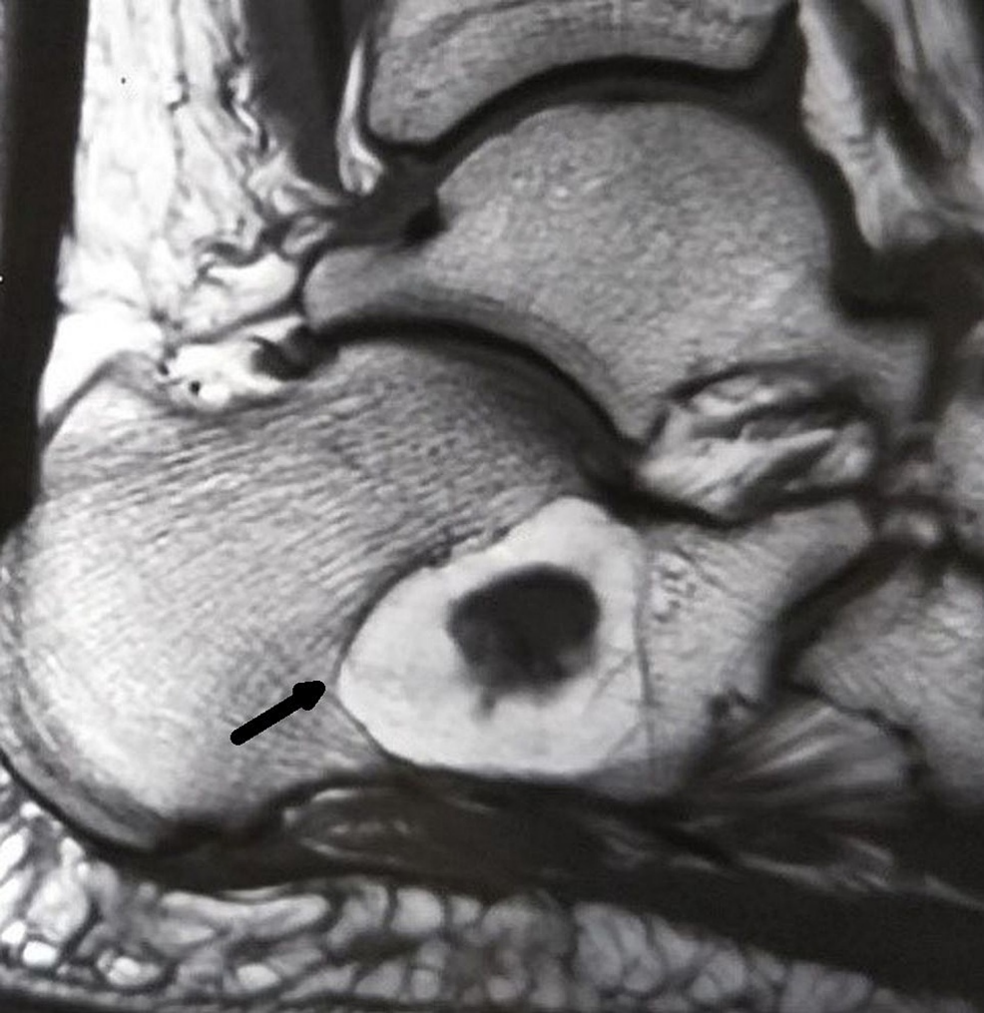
Magnetic resonance imaging of the right ankle at presentation The T1-weighted sagittal image demonstrated a 3 x 2.5 cm lytic lesion in the body of calcaneum (black arrow), with hyperintense signals characteristic of fatty tissue, in addition to minimal effusion in the plantar fascia and an intact calcaneum.
